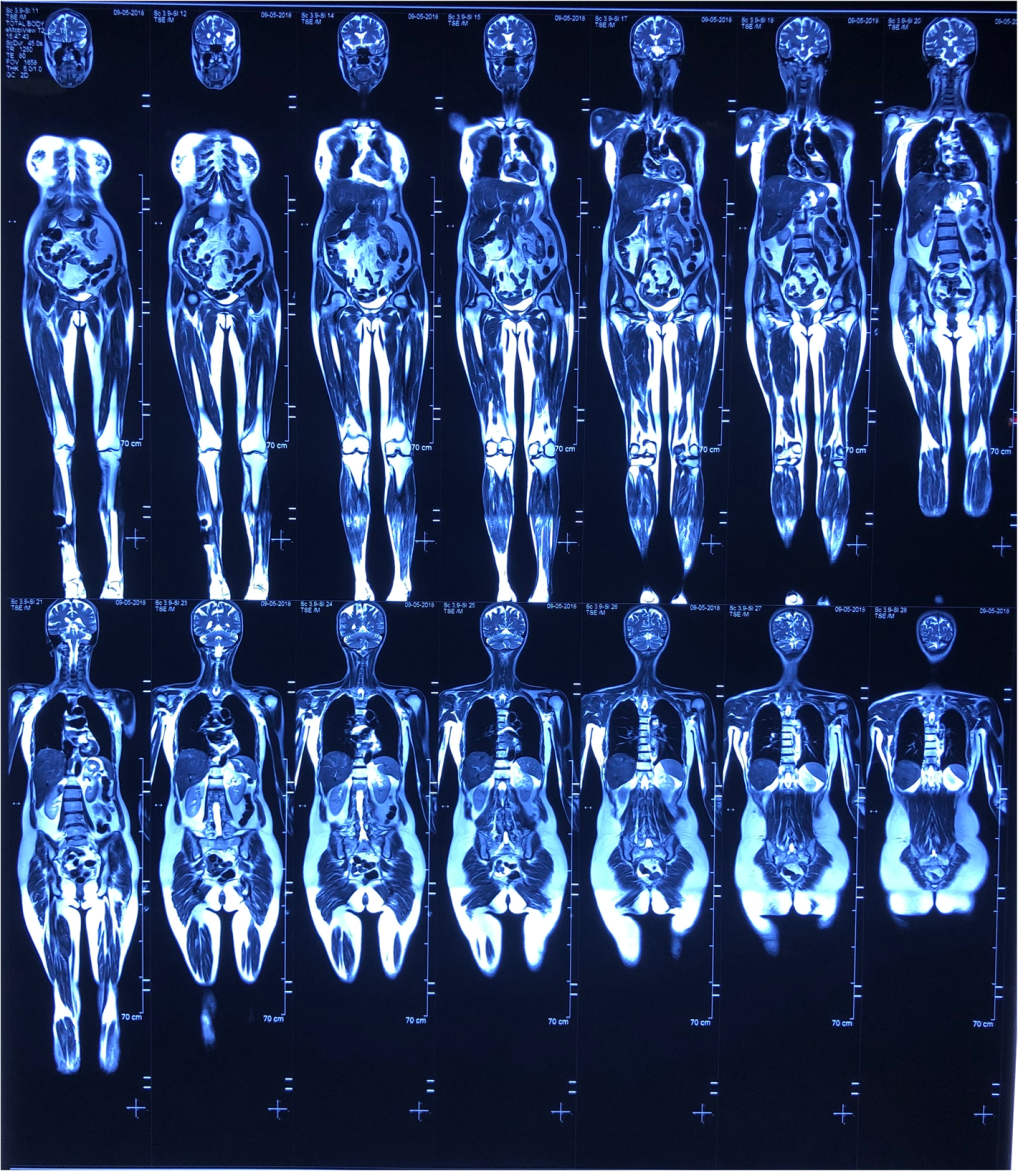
Normal whole-body magnetic resonance imaging
